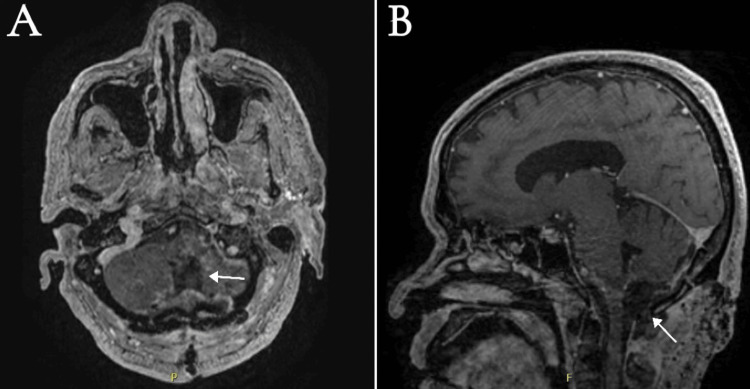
Postoperative postcontrast T1-weighted MRI of the brain: (A) axial and (B) sagittal. The image reveals complete resection of the cervicomedullary junction hemangioblastoma. Posterior fossa postoperative changes with surgical bed mild enhancement and a focal small hemorrhage within the upper part of the medulla (white arrows) can be noted.
